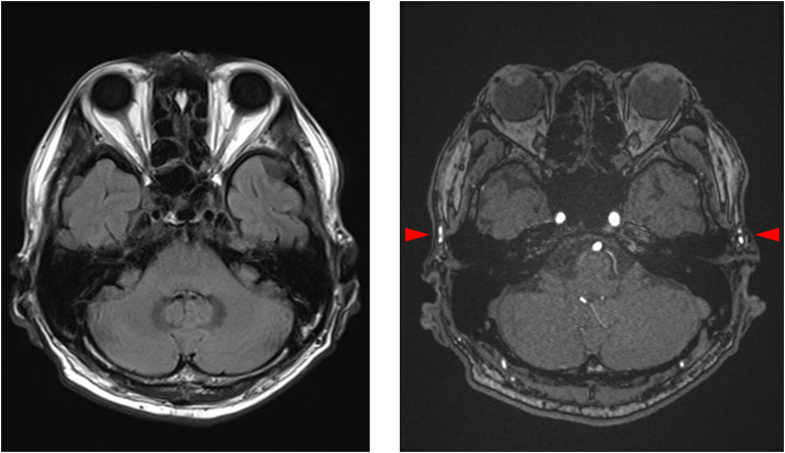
Contrast-Enhanced MRI of the Brain A contrast-enhanced magnetic resonance imaging (MRI) of the brain showed that both temporal arteries were slightly enlarged and displayed some gadolinium enhancement (red arrows).
